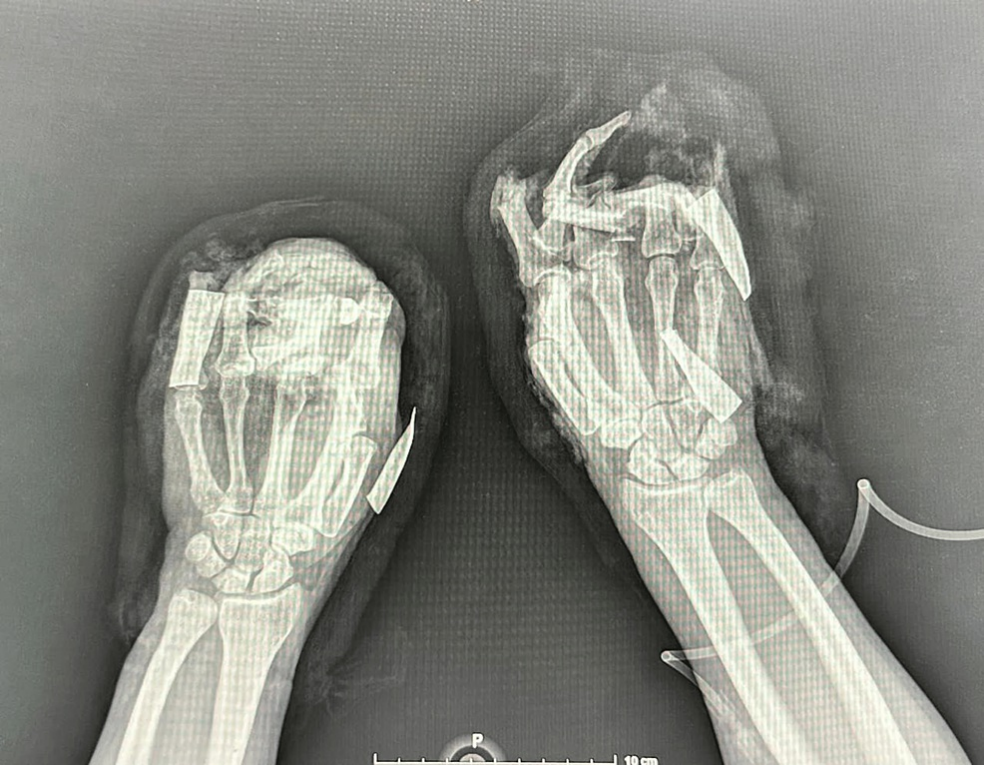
Anteroposterior projection of both hands. This radiographic image reveals the extensive injuries sustained to the right hand, including the loss of middle and distal phalanges of the second finger, and multiple fractures and skeletal disruptions consistent with a crushing mechanism of injury.
